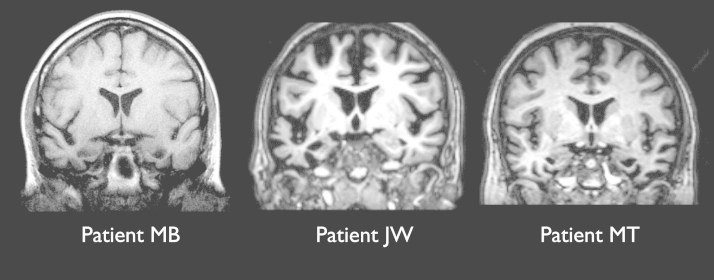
MRI scans for each patient Images are presented in neurological convention (left on left).
